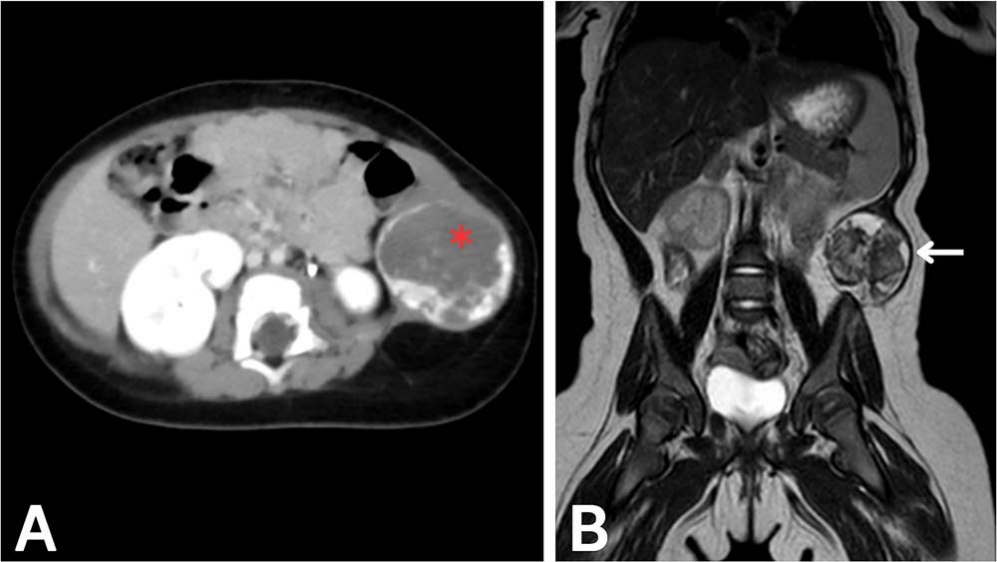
Imaging of the abdominal wall mass. (A) Axial post-contrast CT image in the venous phase demonstrates peripheral centripetal enhancement of the lesion (asterisk). (B) Coronal T2-weighted MRI reveals the anatomical relationships of the mass (arrow).
